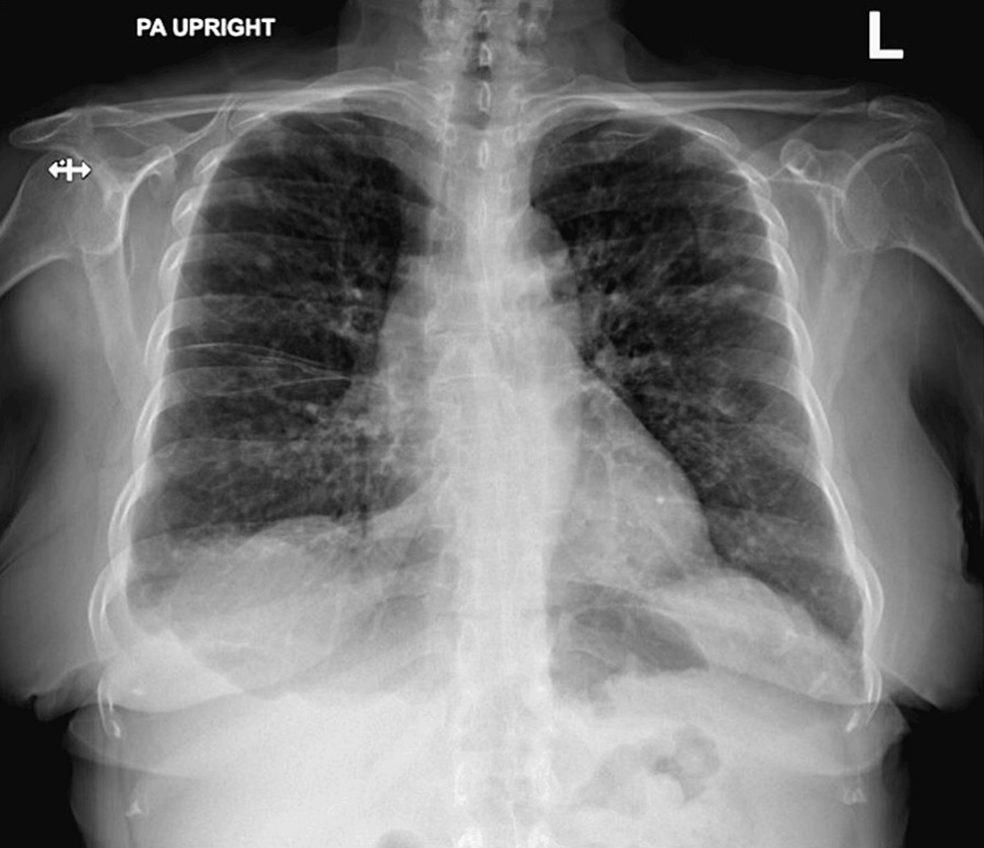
Chest radiography showing a small right pleural effusion and bilateral reticular pulmonary opacities.
